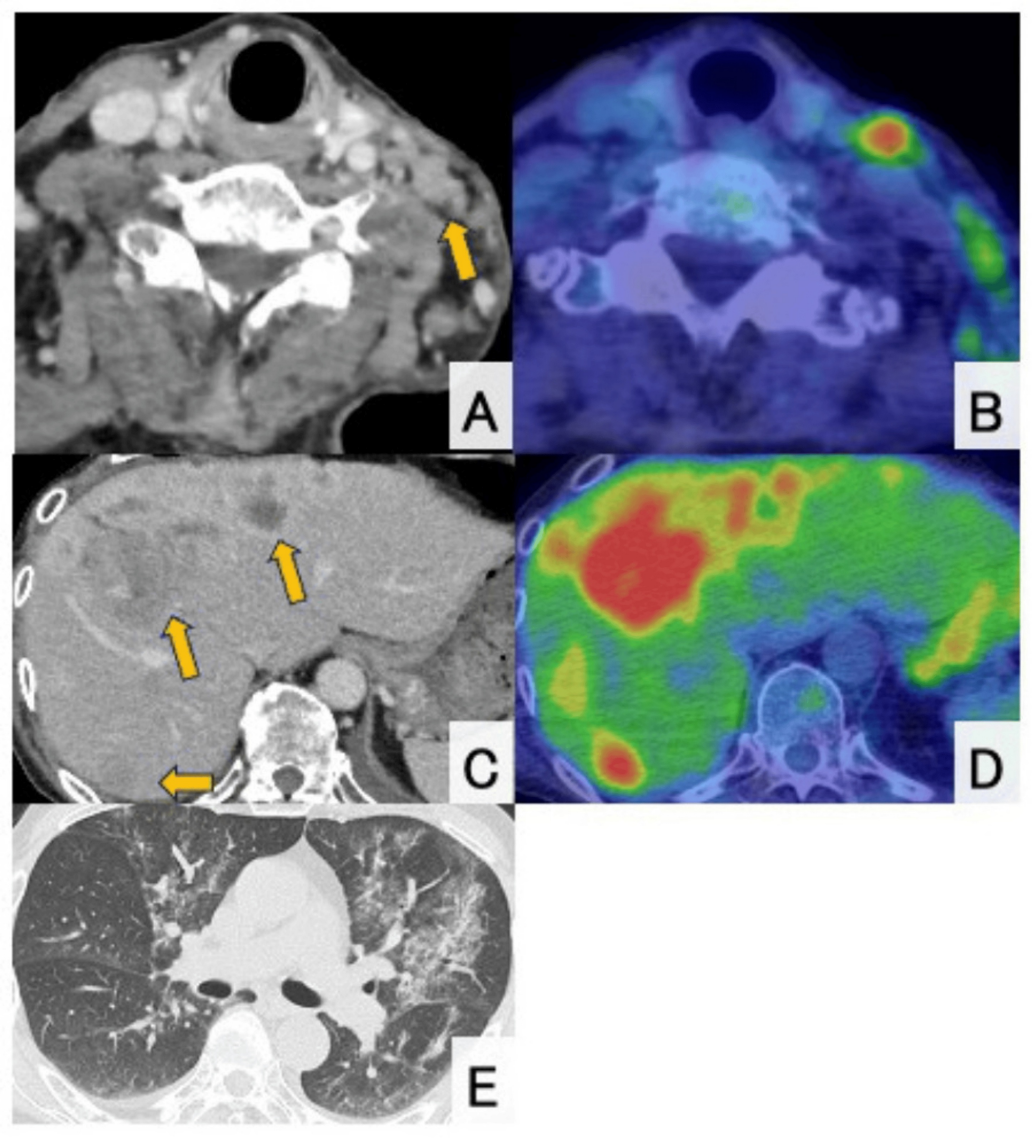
Contrast-enhanced CT and PET findings. (A) and (B) Contrast-enhanced CT and corresponding 18F-FDG-PET/CT images demonstrate multiple enlarged lymph nodes extending from the left cervical region to the axilla, with the largest measuring approximately 32 mm. Notably, no abnormal FDG uptake is observed in the thyroid gland. (C) and (D) Contrast-enhanced CT of the abdomen shows multiple hepatic masses (up to 63 mm) with ring enhancement, which correspond to areas of increased FDG uptake on the PET/CT image. (E) Chest CT shows bilateral ground-glass opacities. PET: positron emission tomography; CT: computed tomography; FDG: fluorodeoxyglucose.
